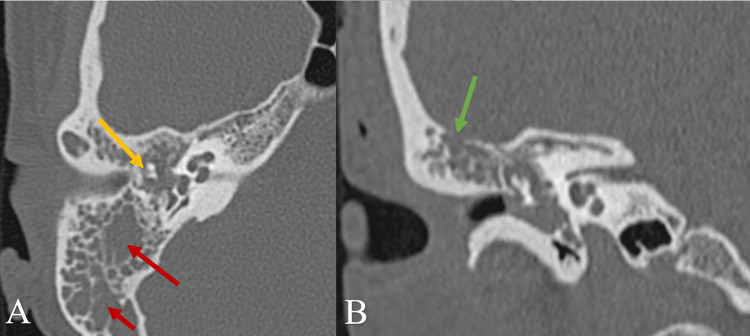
Preoperative axial (A) and coronal (B) CT images of the right ear in 2009 Opacification of the mastoid air cells (red arrows), ossicles embedded but not destroyed by the tumor (yellow arrow), and dehiscence of tegmen mastoideum (green arrow) are seen.
